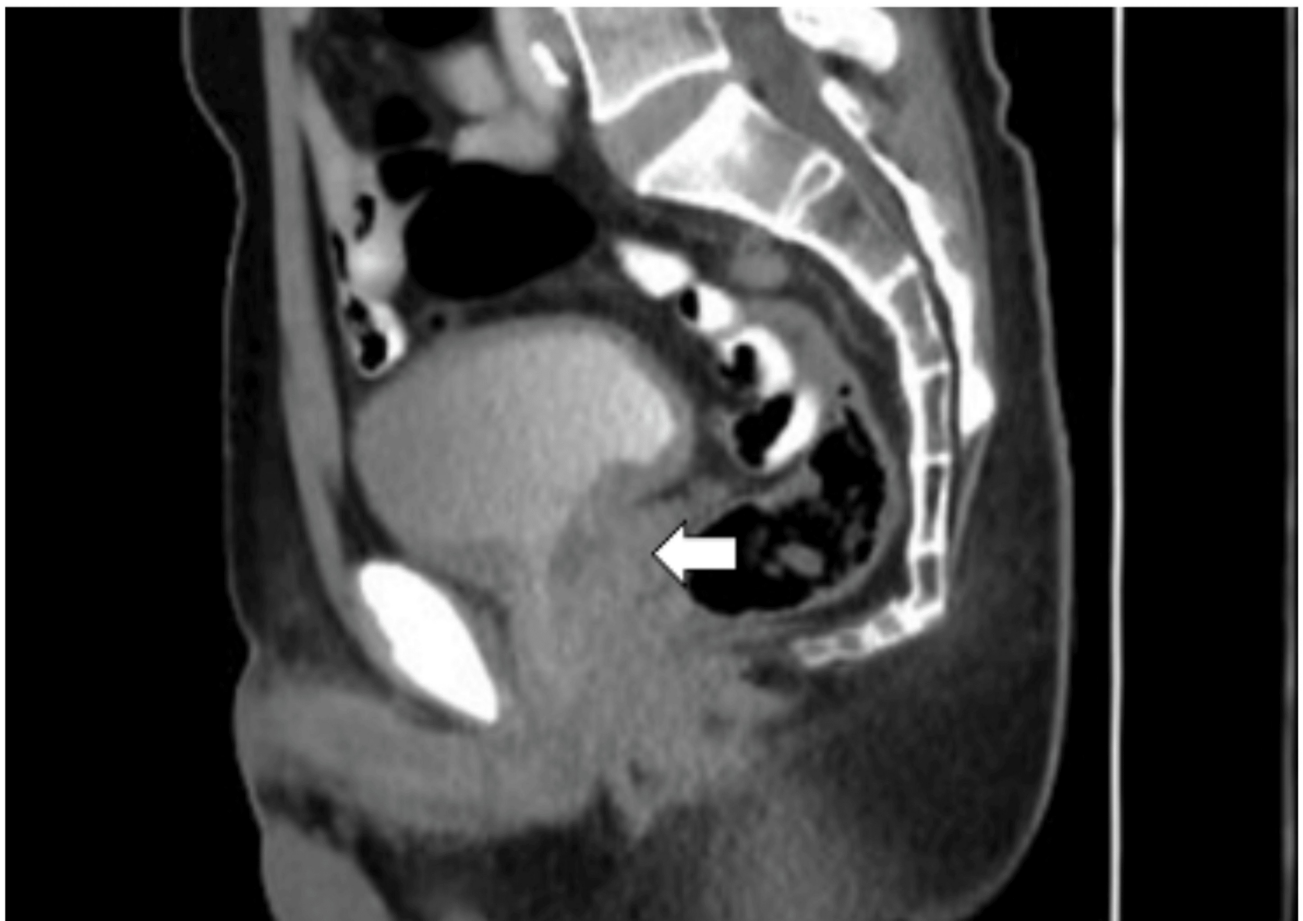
Abdominopelvic multislice computed tomography scan showing infiltrative mass (axial view). The white arrow demonstrates a solid mass involving the posteroinferior bladder wall, perivesical fat, prostate gland, and seminal vesicles.
